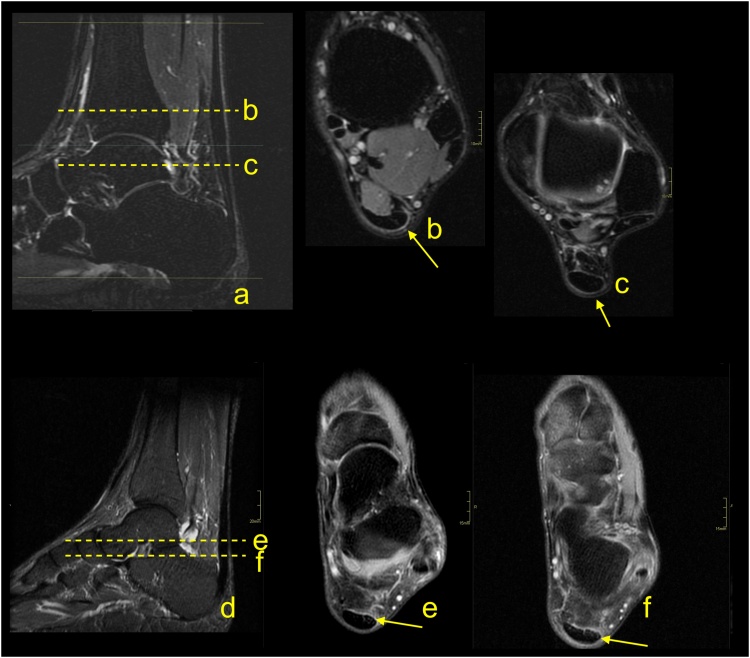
Paratenonitis. Two patients with pain of the Achilles tendon. a–c: a 32-year-old patient with the pain of the Achilles tendon after running. d–f: a 56-year-old patient with pain of the Achilles tendon without training. MRI (T2-weighted with fat suppression) revealed a higher signal of the paratenon (b, c, e and f) with insignificant tendinopathy of the Achilles tendon (a–c).
